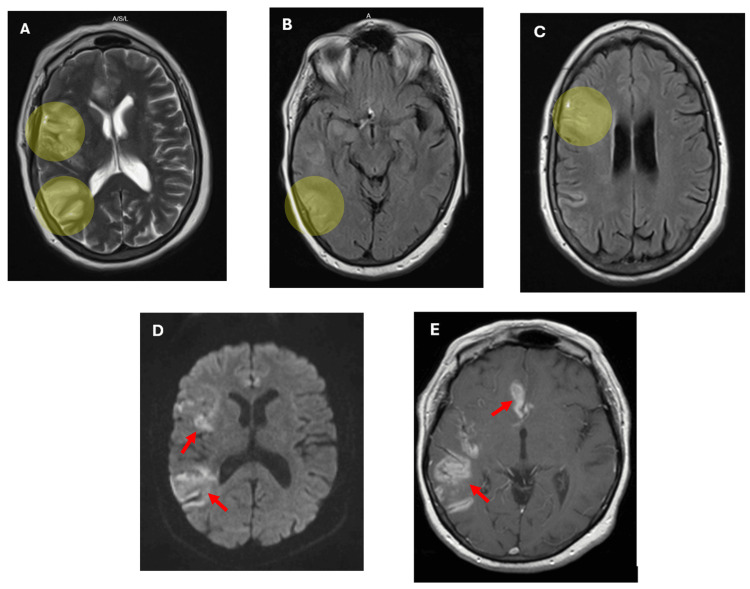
(A) Hyperintensity in the right MCA territory on axial T2-weighted MRI of the brain (yellow highlight). (B) FLAIR hyperintensity in the right temporal lobe on axial FLAIR sequence MRI of the brain (yellow highlight). (C) FLAIR hyperintensity in the right frontal lobe on axial FLAIR sequence MRI of the brain (yellow highlight). (D) Restricted diffusion in the right MCA and ACA territories, involving the right frontal and parietal lobes and the frontal parafalcine area, on DWI B1000 sequence axial MRI of the brain (red arrows). (E) Areas of enhancement in the right frontal and parietal lobes on axial T1-weighted post-contrast MRI of the brain (red arrows). MCA, middle cerebral artery; ACA, anterior cerebral artery; MRI, magnetic resonance imaging; FLAIR, fluid-attenuated inversion recovery; DWI, diffusion-weighted imaging
